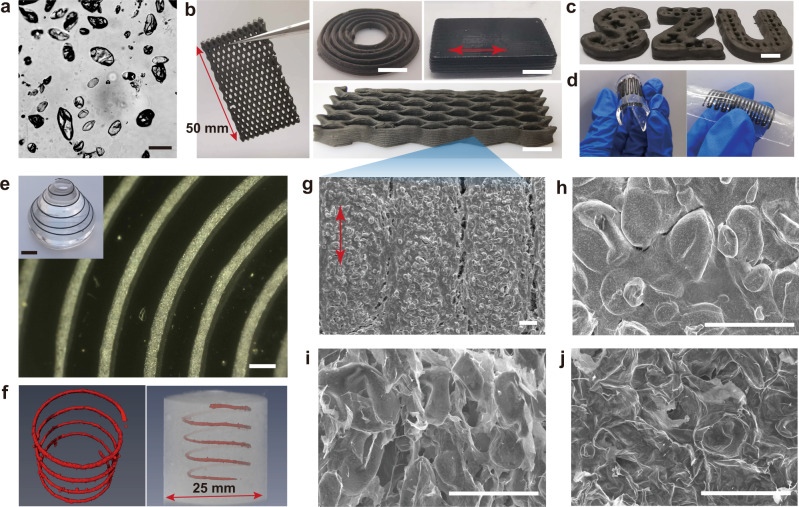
3D printing of PW@NCs. a OM image of GO_1.0%_/DDAB-PW emulsion droplets. b–d Optical images of various 3D-printed structures (b, c) and soft bent substrates with zigzag NCs embedded in d. e OM and optical images of the printed isometric spiral on the out-of-plane substrate. f Reconstructed 3D X-ray microcomputed tomography of the printed spiral in cement. g–i SEM surface (g, h) and cross-sectional images of PW@NC (g). j Cross-sectional SEM images of PW@NCs after annealing at 80 °C for 10 min. Scale bars, 100 μm (a), 5 mm (b, c, inset in e), 500 μm (e, g), 50 μm (f–h).
